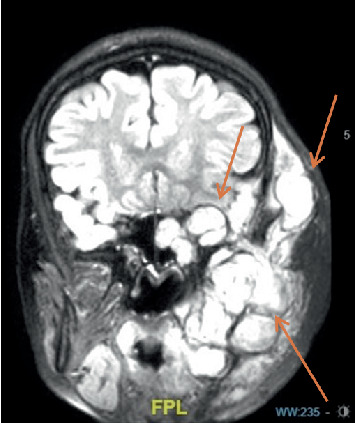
Magnetic resonance imaging (MRI) taken 1 month after titanium mesh removal showed extensive lesion progression with infiltration of the left parapharyngeal, left carotid, and left cranial extension.
